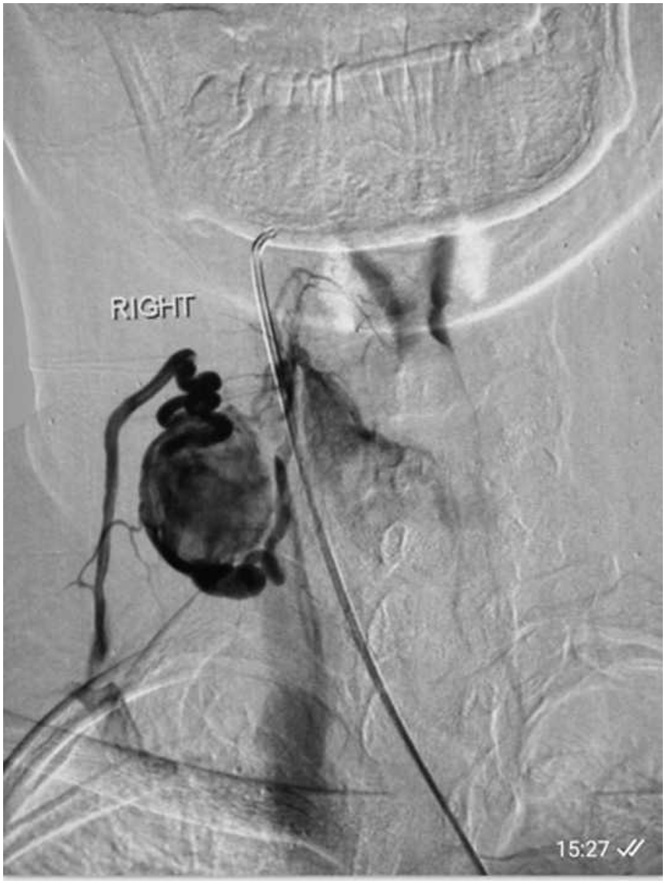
Selective right external carotid angiogram showing highly vascular fistulous type of lesion fed by superior thyroidal artery and draining into internal jugular vein corresponding to vascular lesion visible on CT neck giving rise the suspicion of thyroid paraganglioma.
